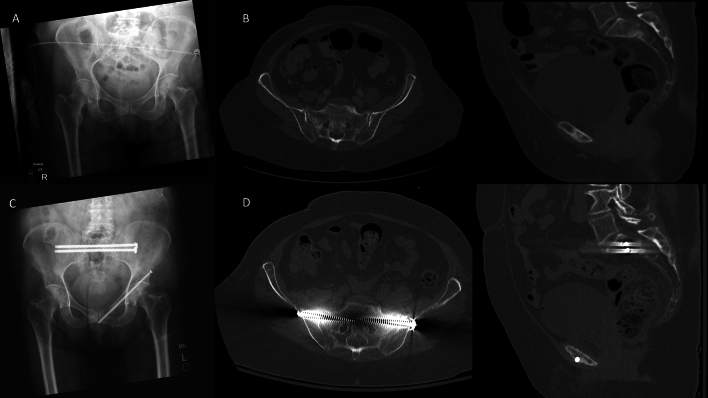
U-type sacral fracture with minimal displacement treated with percutaneous sacral screws. A Injury radiographs. B On axial and sagittal CT cuts, minimal displacement is noted, and osseous fixation pathways preserved for multiple trans-iliac trans-sacral screws in the upper sacral pathway. C Postop radiographs. D Postoperative CT
